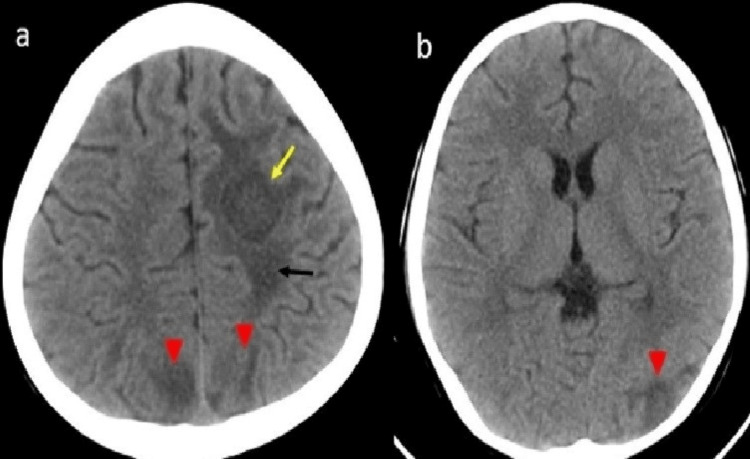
Non contrast CT Brain after new onset seizure showing (a) Iso to hypodense left centrum semiovale subacute hemorrhage with mild edema (b) Newly developed ill defined hypodensities involving cortex and sub-cortical regions in bilateral high parietal and left tempero-occipital lobe suggestive of acute infarcts.
